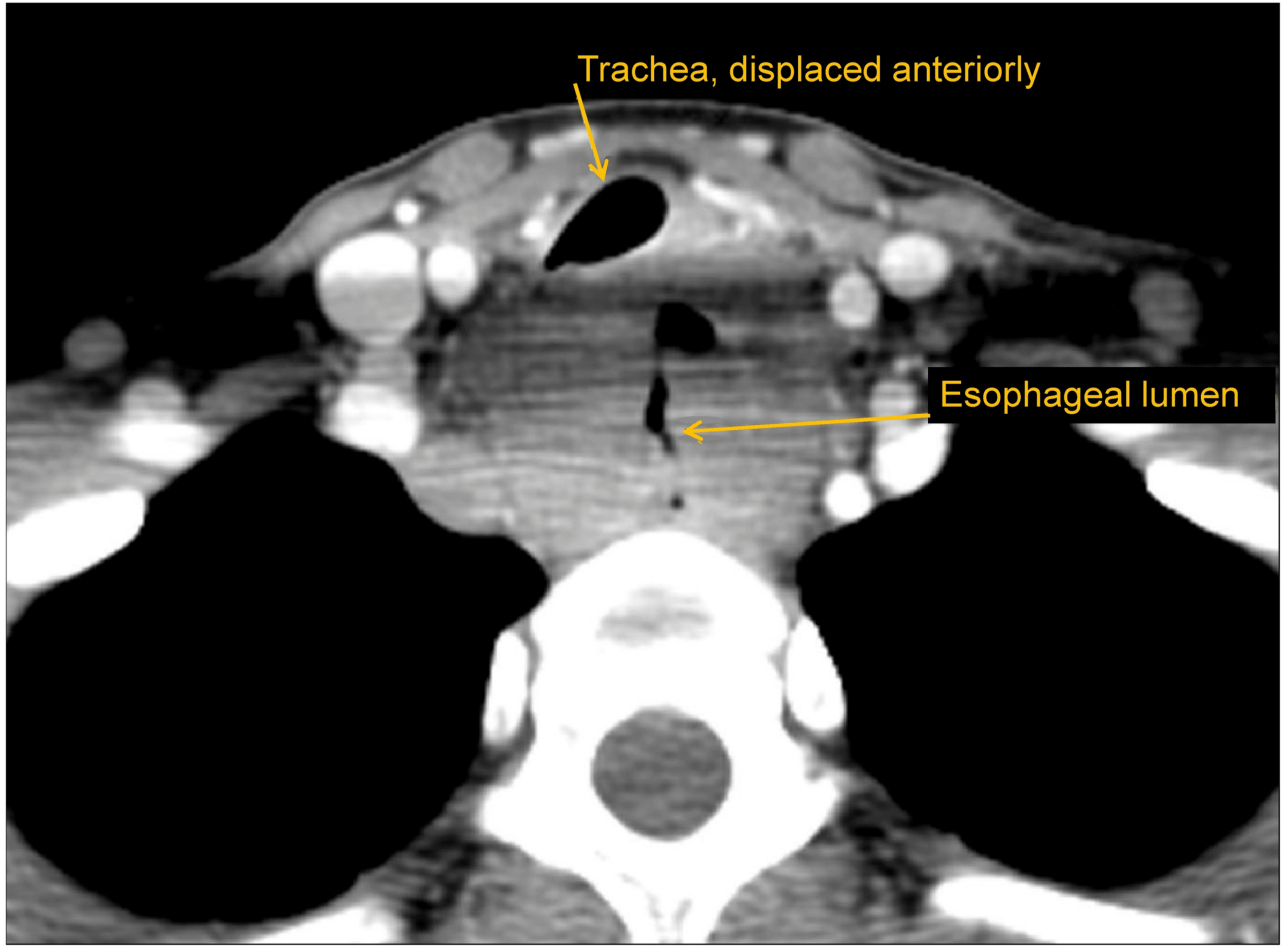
Axial view of neck CT of a 24-year-old with dyspnea and dysphagia, showing large neoplasm involving the esophagus, resulting in tracheal displacement and compression (Case 2)
